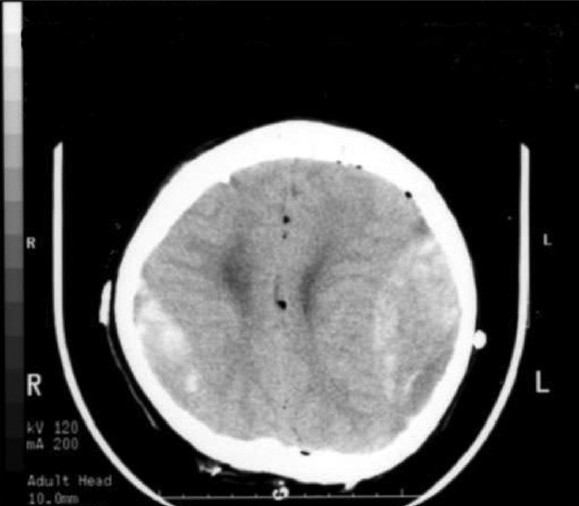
Immediate postoperative brain CT showing acute EDHs in bilateral frontoparietal regions
